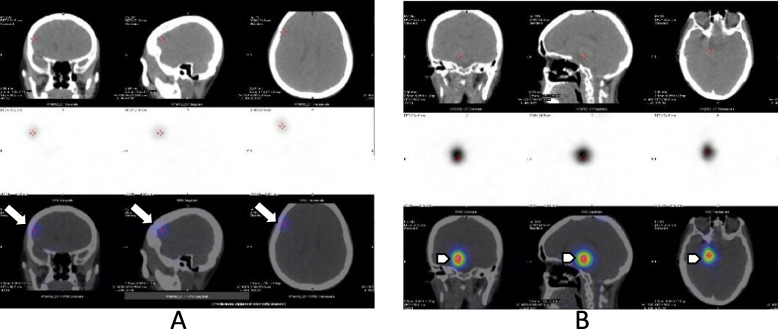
2 mCi.^131^I-SPECT/CT head and neck (a): Mild metastatic focal uptake localized in the right frontal lobe (arrow) (b): High metastatic focal uptake localized in the right pedicular (arrow)
